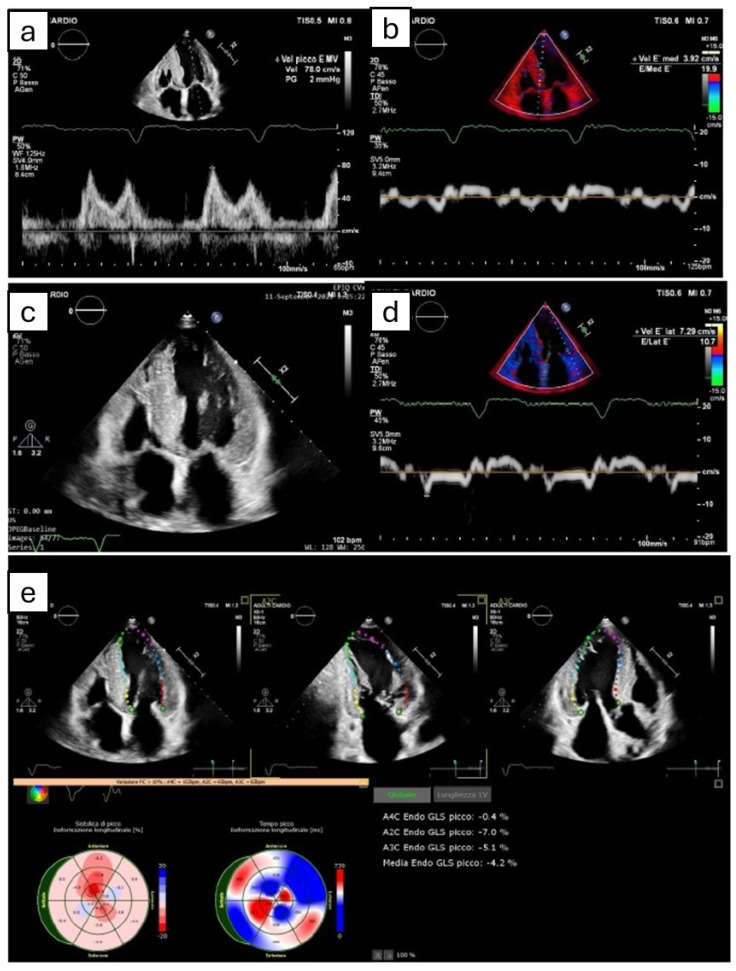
The figure shows the most common echocardiographic signs that can potentially suggest amyloidotic cardiomyopathy, even though none of them are pathognomonic. (a,b,d) Grade II diastolic dysfunction, with pseudonormal pattern at transmitral power Doppler (a) and reduced septal (b) and lateral (d) e’ velocity at tissue Doppler imaging. (c) Symmetric biventricular hypertrophy, with thickening of interatrial septum. (e) Apical sparing pattern derived by Speckle tracking echocardiography.
